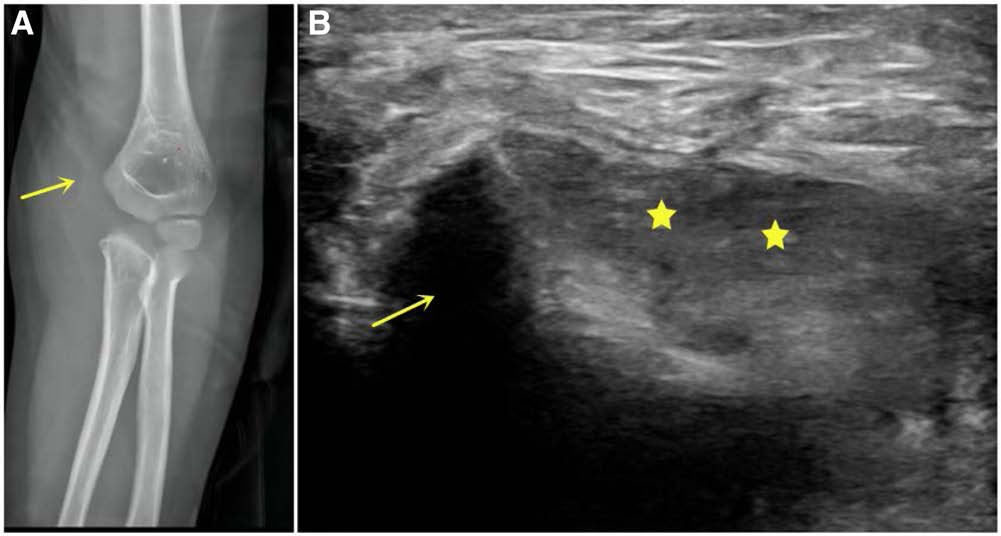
(A) A 5-yr-old boy, X-ray image showed swelling of the medial soft tissue and a thin “C” shaped fracture fragment (yellow arrow) was visible. (B) The transverse ultrasound scan showed that the cartilage of medial condyle was displaced (yellow arrow) from cartilage hinge of distal humer (yellow star).
